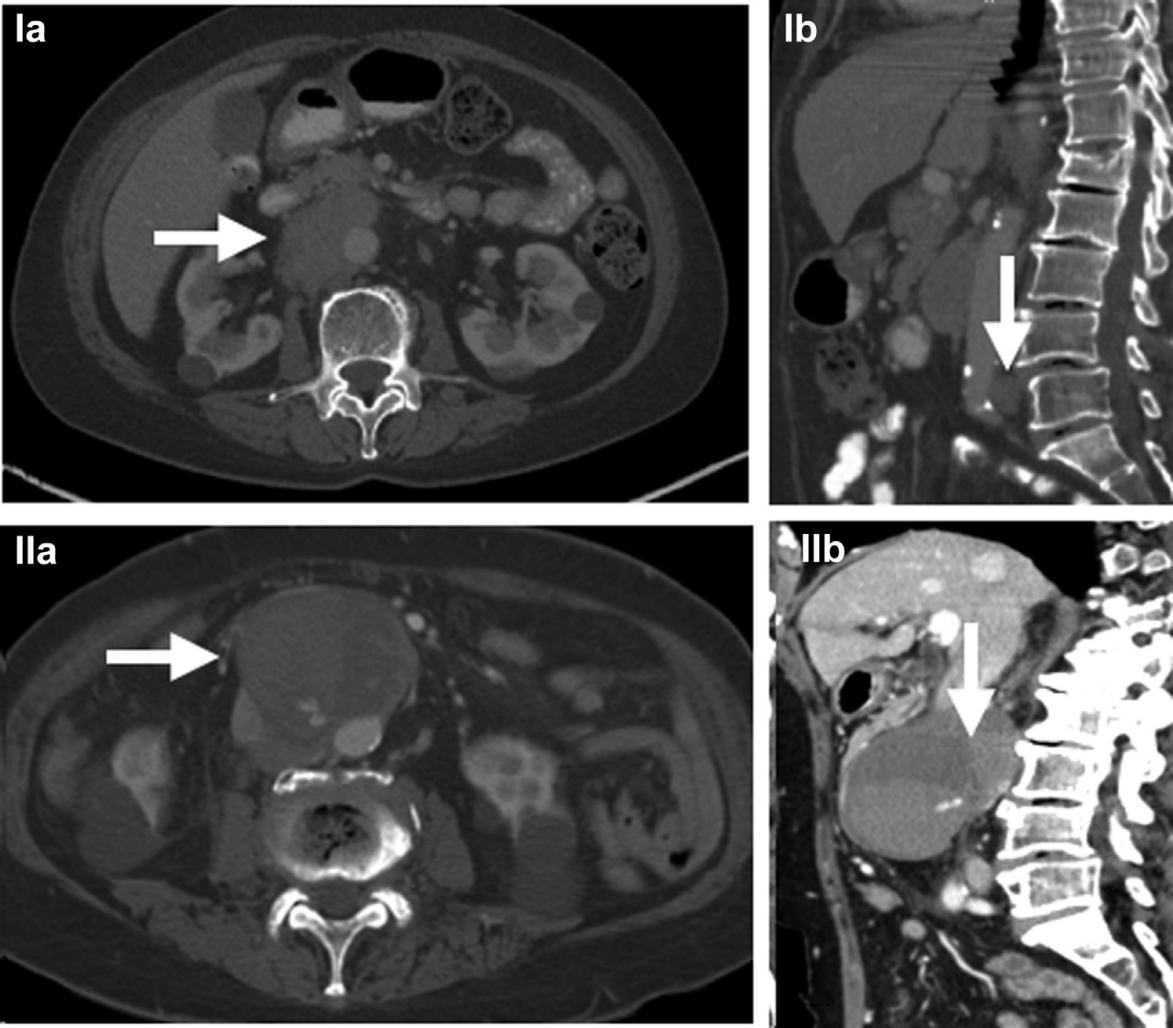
Computed tomography angiograms before rupture from 2019 with evidence of mild lymphadenopathy, in transverse (Ia; *arrow*) and coronal (Ib; *arrow*) views. Computed tomography angiograms after rupture showing evidence of significant lymphadenopathy with infrarenal aortic pseudoaneurysm and active bleeding, in both transverse (IIa; *arrow*) and coronal (IIb) views. Lymphoma (*arrow*) can be differentiated from rupture in IIa.
